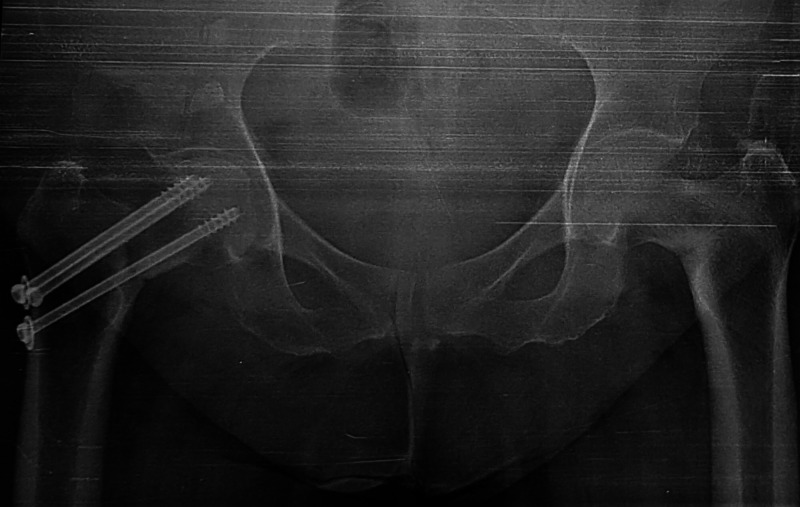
Anteroposterior both hips radiograph with right femoral neck fracture and initial screw fixation in situ with coxa-vara of left hip
